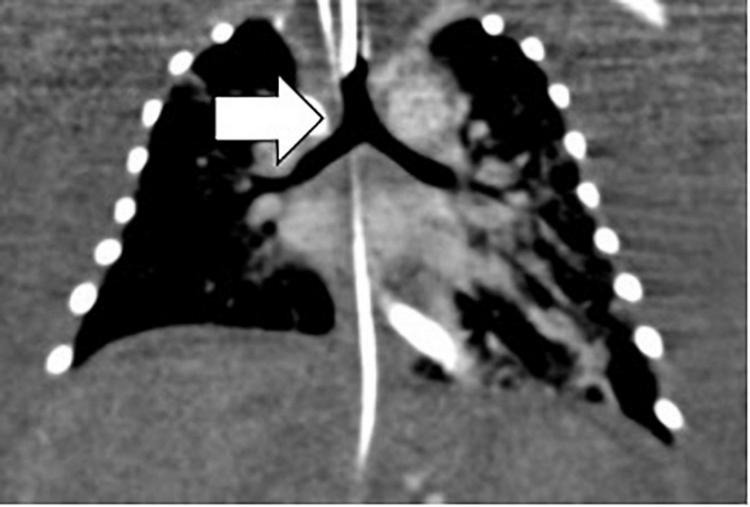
Bronchial tree anatomy and the sub-type of isomerism by neonatal thoracic CT. Arrow white, left isomerism of tracheal bifurcation with bi-lobar lungs.
